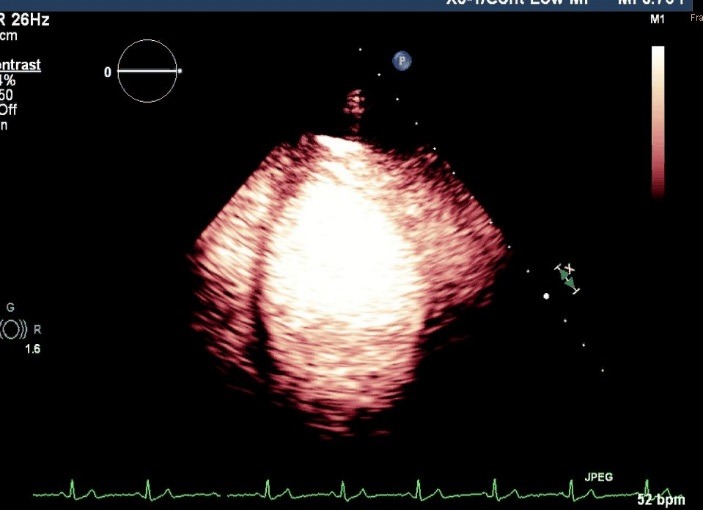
Contrast echocardiography, 4-chamber view - absent perfusion in the lower basal septum and heterogeneous uptake of the mid-third and apical segments (personal archive)
